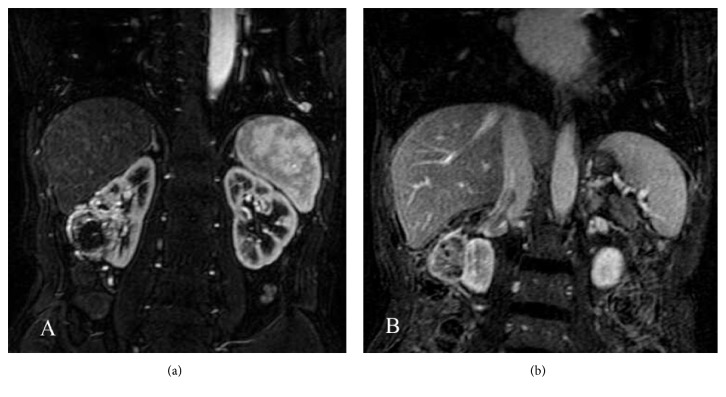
(a) Abdominal MRI demonstrating right renal cell carcinoma (arrow). (b) MRI also demonstrating tumor thrombosis in the inferior vena cava.
